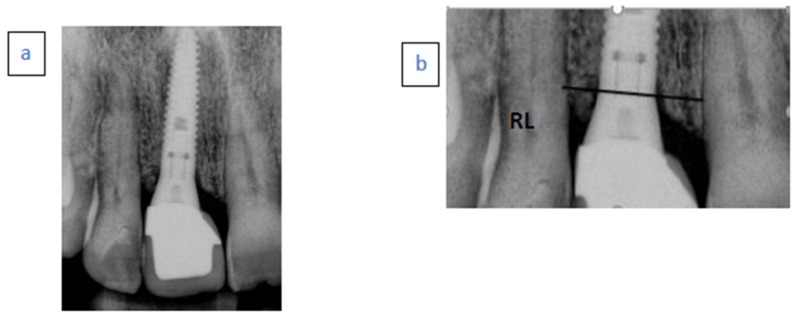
(a) Peri-apical radiograph 45 months after definitive crown placement. (b) Reference level (RL)was used to determine crestal bone level (CBL) values.
